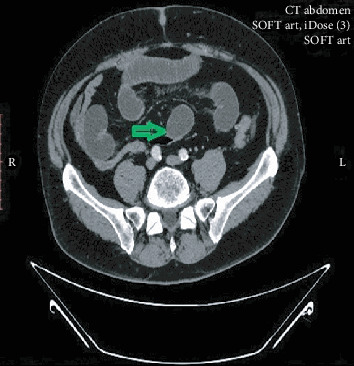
CT scan of the abdomen—green arrow showing the transition point in the terminal ileum, adhered to the sigmoid colon.
